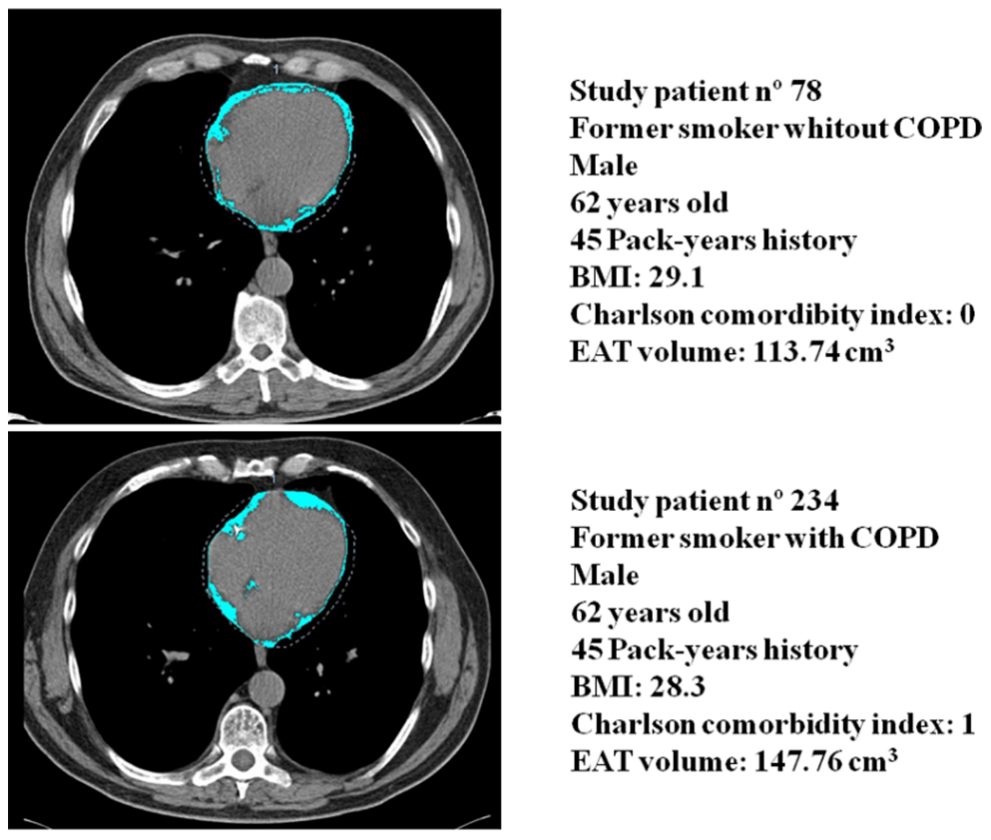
CT of two matched patients participating in the study showing their EAT volume measurements in clear blue.
